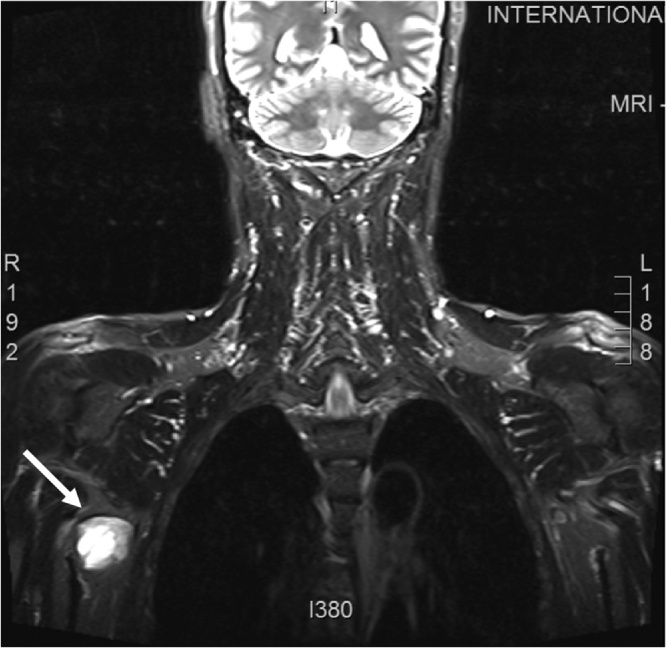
MRI of the right axillary area involved, showing a lesion in the right axilla measuring 3.0 × 3.8 × 2.3 cm, with primary cystic component and irregular thickened wall.
